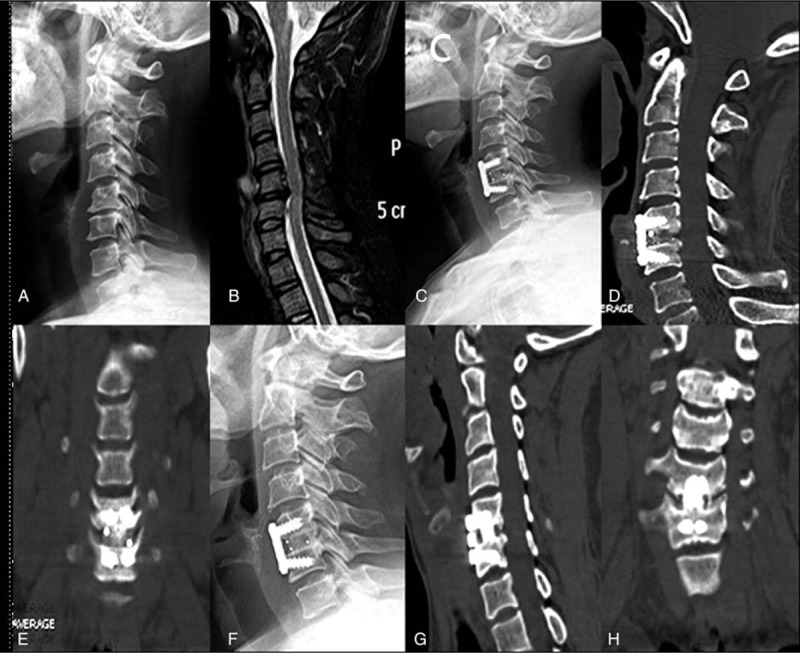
The graph shows a case using PEEK cage for ACDF. There is bony lucency at the juncture of the cage and vertebralbody on CT 3 months after operation, while which disappear at the time of 6 months after operation. A 47-year-old woman with cervical spondylotic myelopathy. (A, B) preoperative lateral X-ray films and preoperative magnetic resonance imaging (MRI); (C-E) X-ray 3 months after operation and CT 3 months after operation; (F-H) X-ray 6 months after operation and CT 6 months after operation.
